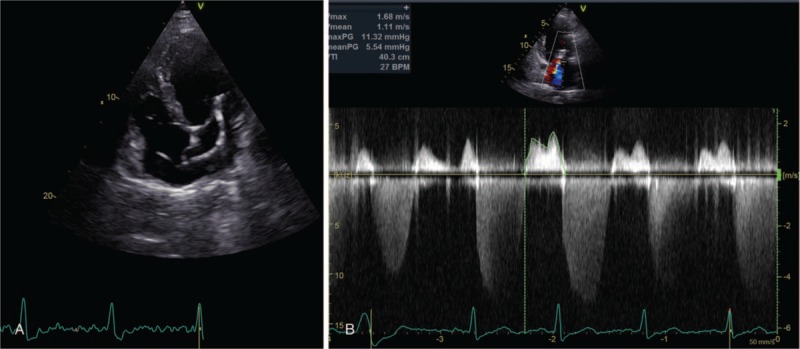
A, Four-chamber 2D echocardiographic view showing defibrillator lead placed in the left ventricle after its passage through an iatrogenic atrial septal defect. B, Echocardiographic 2D Doppler view displaying an accelerated transmitral diastolic flow. 2D = two-dimensional.
